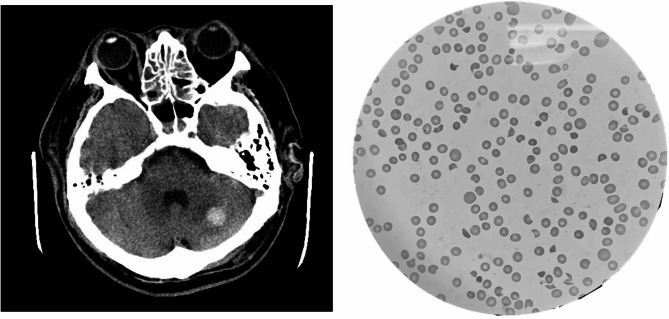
(a) Brain computed tomography of left cerebellum intracerebral hemorrhage; (b) Peripheral blood smear of schistocyte
